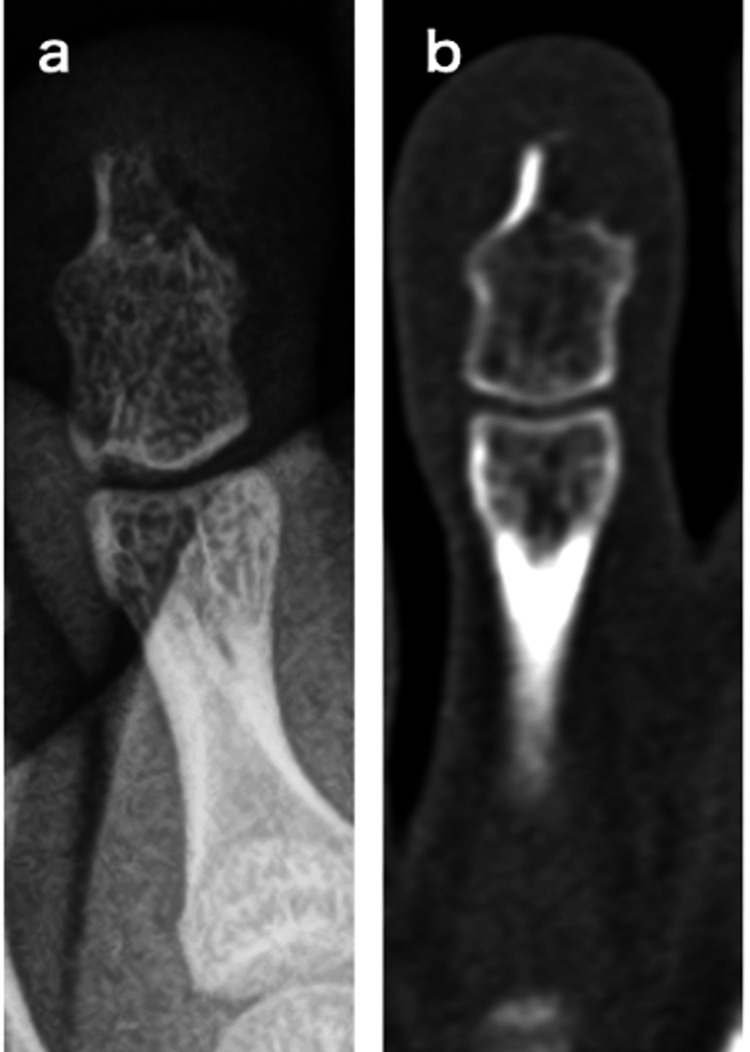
Postoperative images. Postoperative X-ray (a) and CT (b) images.
